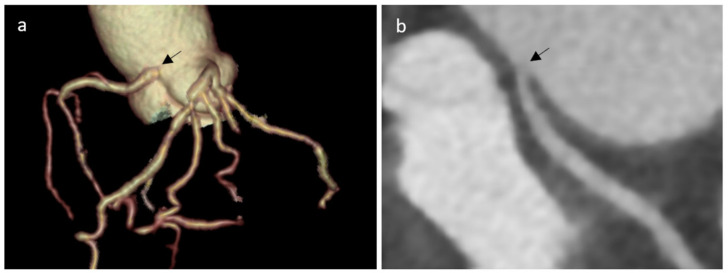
Right coronary artery from left sinus ((a) volume-rendering image, arrow) with slit-like ostium ((b) axial image, arrow) seen by computed tomography.
